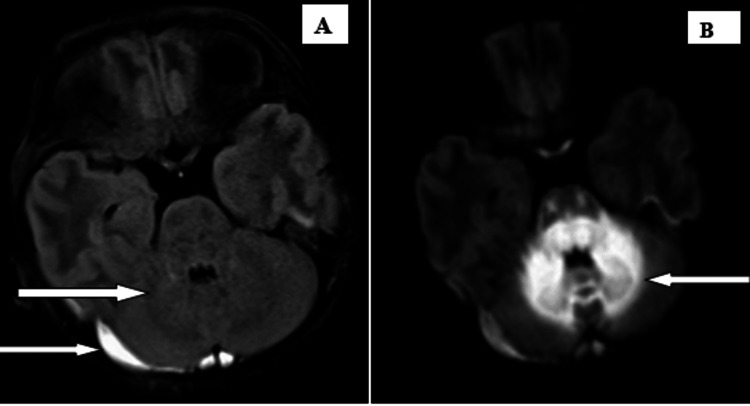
Subtle FLAIR hyperintense are signals noted in the optic chiasma, pons, vermis, and cerebellum. Mild subdural collection is noted in the bilateral cerebellar hemisphere (A). Diffusion restriction is noted in the optic chiasma, pons, vermis, and cerebellum (B). FLAIR: fluid-attenuated inversion recovery
